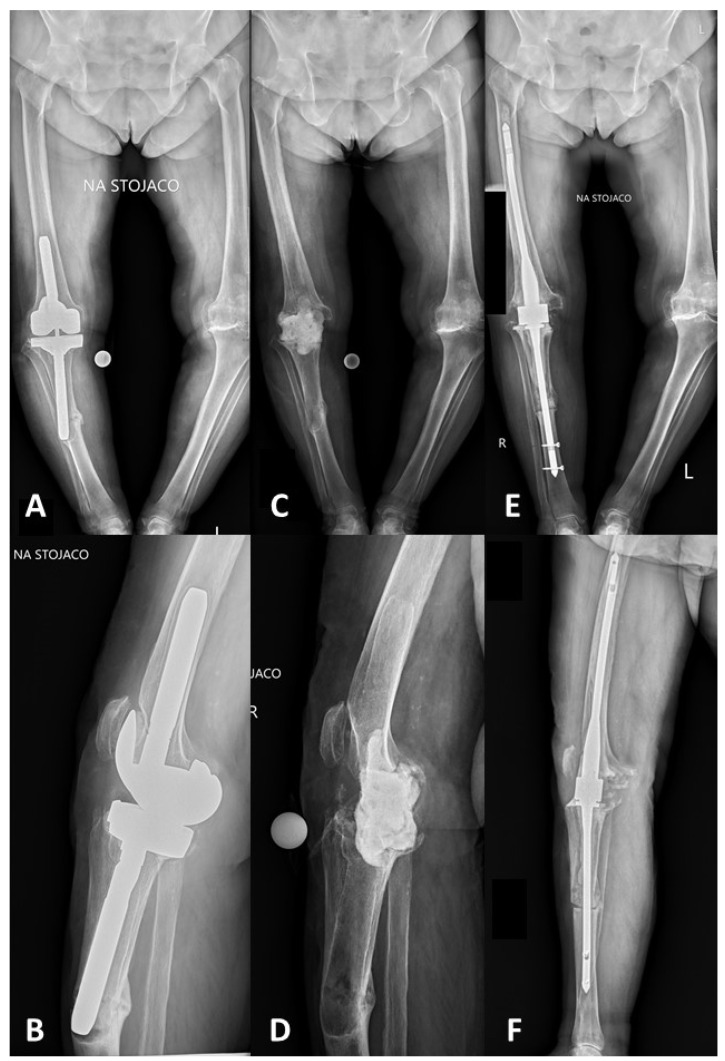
Radiographs of the patient (patient no. 1 in Table 1) with confirmed fungal PJI caused by *Candida parapsilosis*. (A) Preoperative AP long-standing radiograph and (B) lateral knee radiograph. (C) Long-standing AP and (D) lateral radiographs after revision surgery and static spacer implantation contained antibiotics. (E) Long-standing AP and (F) lateral radiographs after knee arthrodesis using a dedicated Charfix2 FN intramedullary nail (ChM, Lewickie, Poland).
